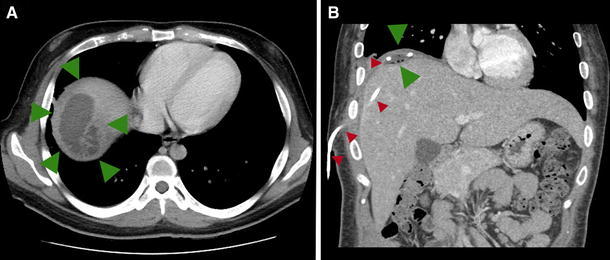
Abdominal CT-scan (a) showed a heterogeneous fluid filled mass in the right hepatic lobe, with thickened internal septations and partial thickening of the outer wall (*green arrows*). The mass measured approximately 7.3 × 5.3 × 4.6 cm^3^. The largest pocket of fluid measured approximately 6.1 × 3.3 cm^2^. Percutaneous drainage of the mass confirmed it to be a pyogenic liver abscess due to *Klebsiella pneumoniae*. After drainage of the abscess and treatment with intravenous antibiotics, (b) the abscess cavity abutting the diaphragm was almost completely cleared (*green arrows*), as shown with the drain in place (*red arrows*)
